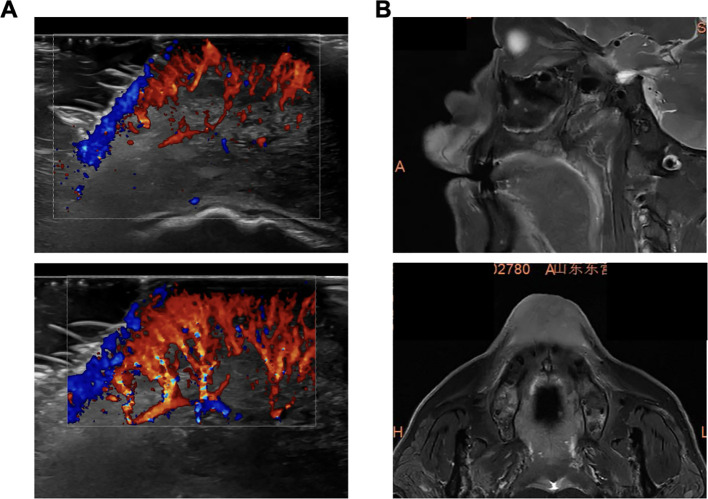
Representative images of ultrasound and MRI examinations. (A) Ultrasound examination revealed a hypoechoic mass with irregular borders and no clear demarcation from surrounding tissues. (B) MRI imaging showed an ill-defined lesion with a slight enhancement.
